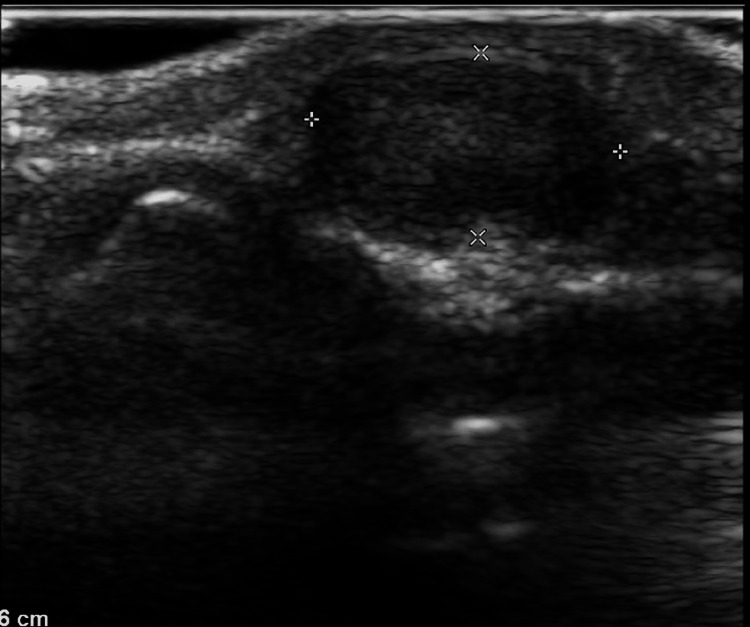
Ultrasound examination of the tumor Ultrasound examination revealed a 9×5 mm cyst, with turbid content, adjacent to the distal phalanx with no internal perfusion or affection of the underlying bone. There was no evidence of increased surrounding vascularity.
